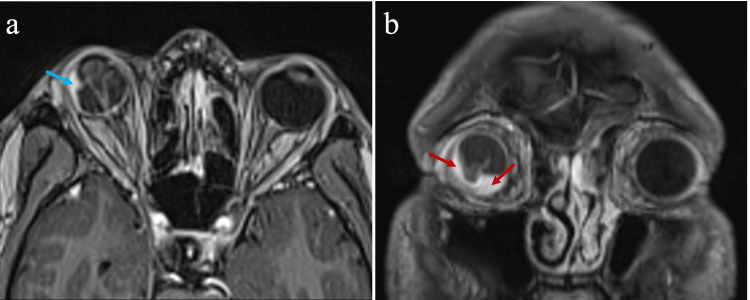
T1 weighted MRI (a) axial section showing enhancing lesion at the temporal region of the right globe (cyan arrow). (b) Coronal section revealed the lesion extending from the temporal to the inferior region of the right globe (red arrow). MRI: magnetic resonance imaging.
